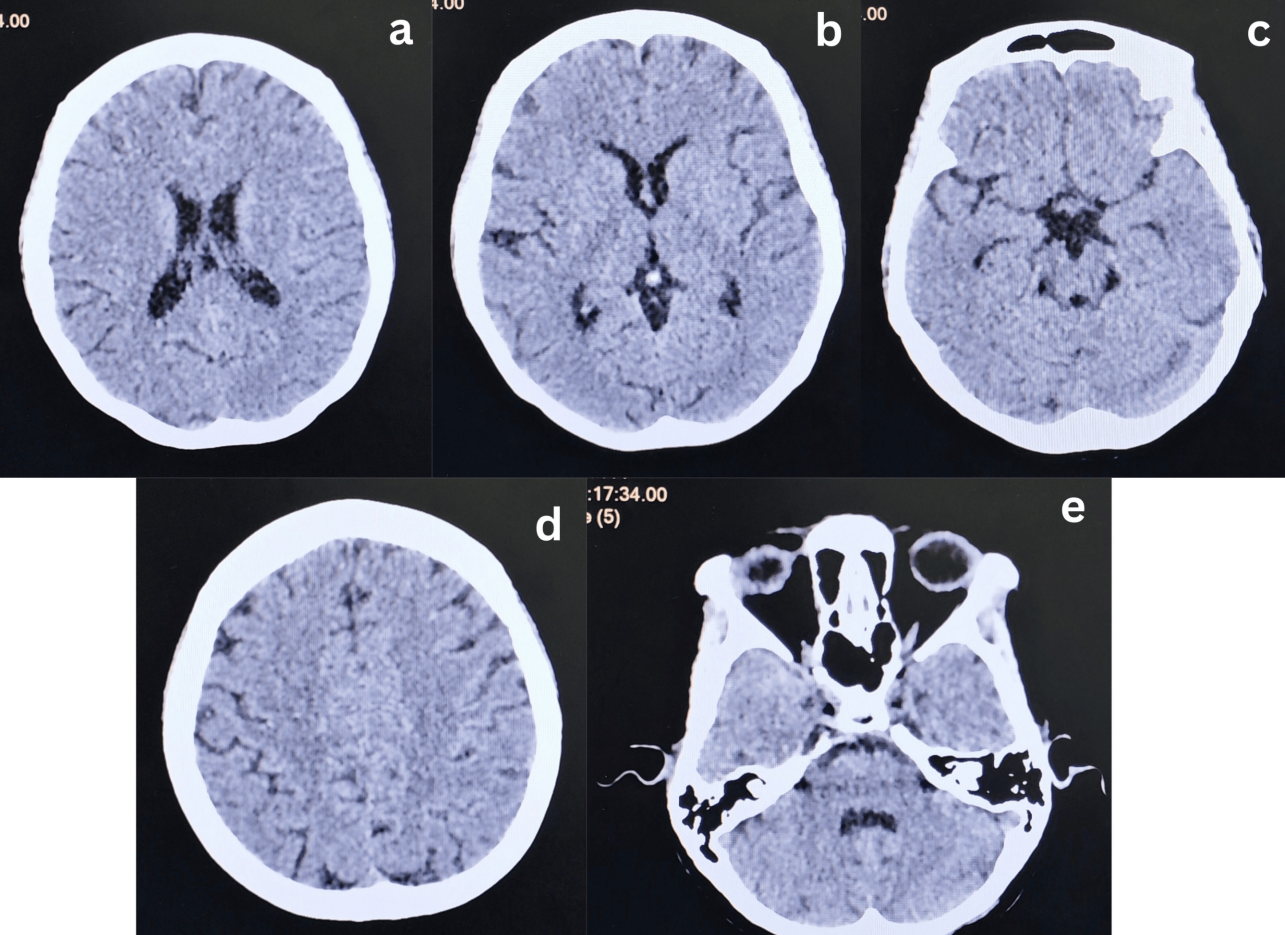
Axial non-contrast CT brain showing no acute hemorrhage, infarction, or mass effect. Normal appearance of the ventricles and cerebral structures. Findings are non-specific. a: Level of corona radiata, b: level of basal ganglia and 3rd ventricle, c: level of basal cistern, d: level of centrum semi-ovale, e: level of 4th ventricle and cerebellum
